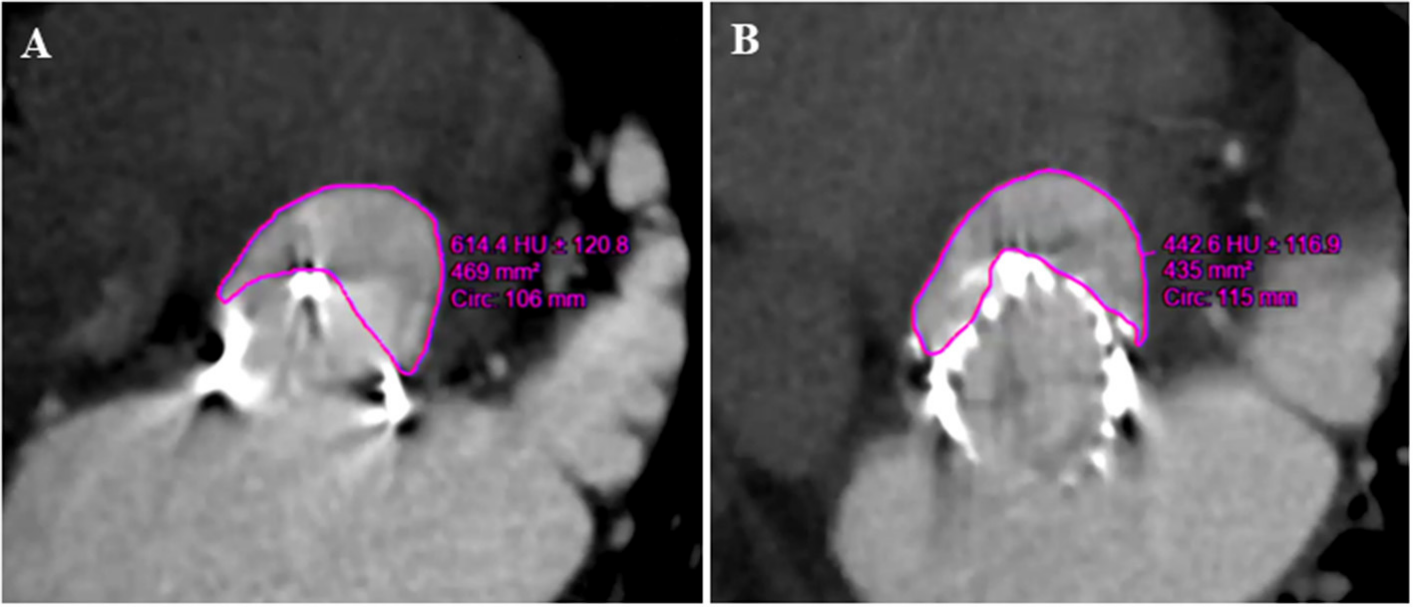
Preoperative and postoperative multidetector CT in the assessment of neo-LVOT (Patient number 23). (A) The predicted area of neo-LVOT was 469 mm^2^ before the J-Valve implantation. (B) The postoperative area of neo-LVOT was 435 mm^2^. LVOT, left ventricular outflow tract.
